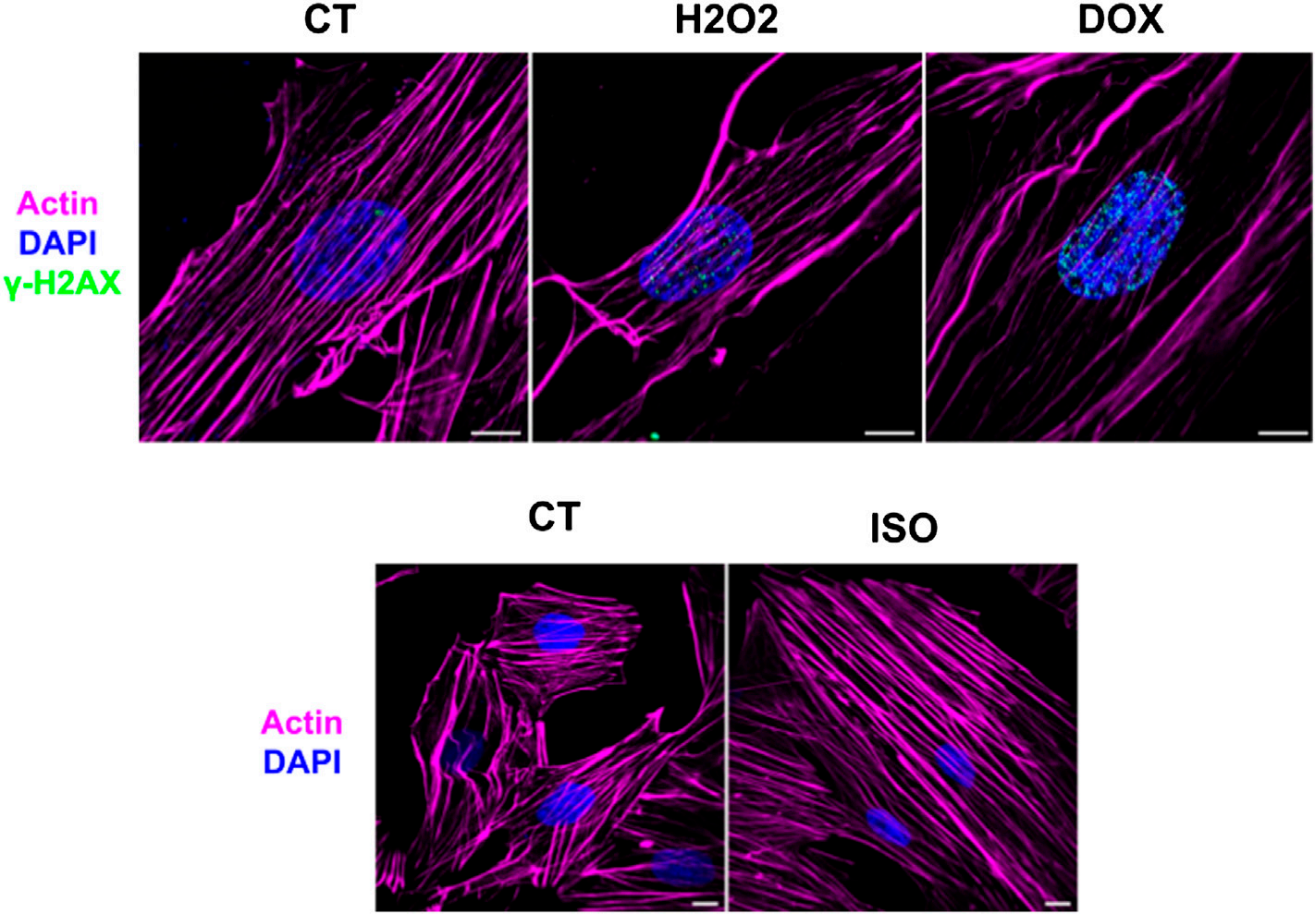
Super-resolution images from control (CT), H_2_O_2_ doxorubicin (DOX) and isoproterenol (ISO) treated H9c2 cardiomyocytes. The efficacy of the oxidative stress resulting from H_2_O_2_ and dox treatment was demonstrated by the increase of the γ-H2AX loci and the effect of isoproterenol was attested by the hypertrophy phenotype of H9c2 cells. Magenta: actin; Blue: nucleus; Cyano: γ-H2AX. Scale bar = 10 µm.
